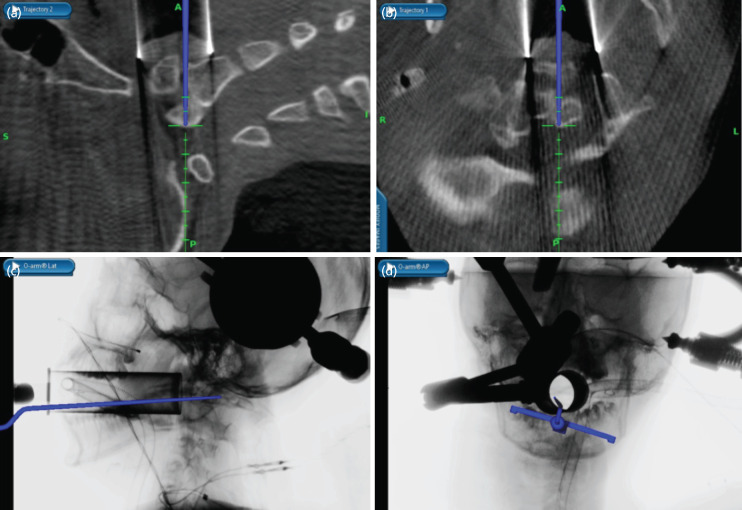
Intra-operative navigation used to identify the tip of odontoid. (a, b) shows the identification of odontoid with the use of passive probe in the sagittal and axial views, respectively. (c, d) Shows the position of the tube and passive probe in AP and lateral radiograph taken by the O-arm.
